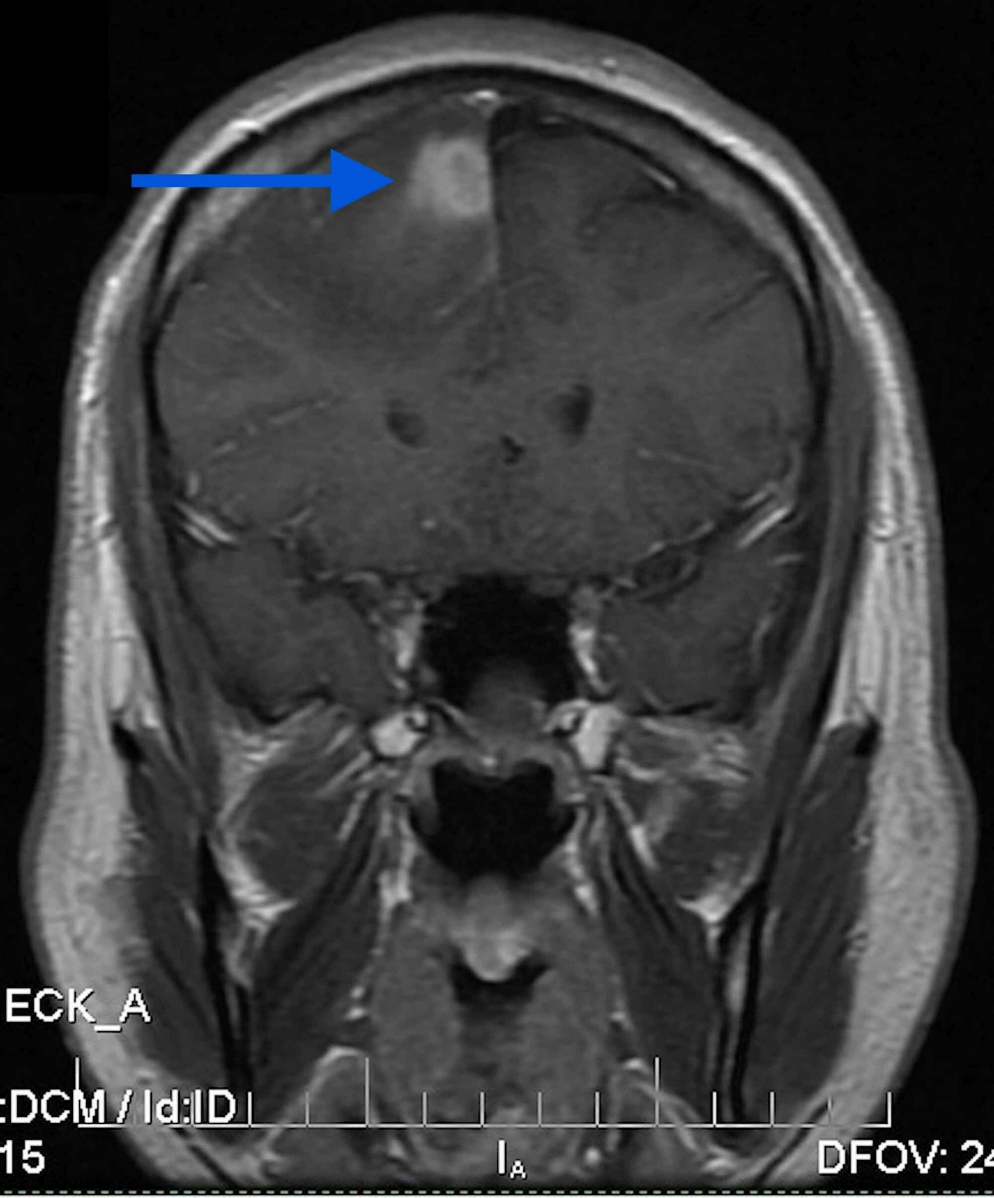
Coronal post-contrast T1 MRI showing 16×14×21 mm conglomerate ring-enhancing lesion in the paramedian region of the right frontal lobe with surrounding edema.
